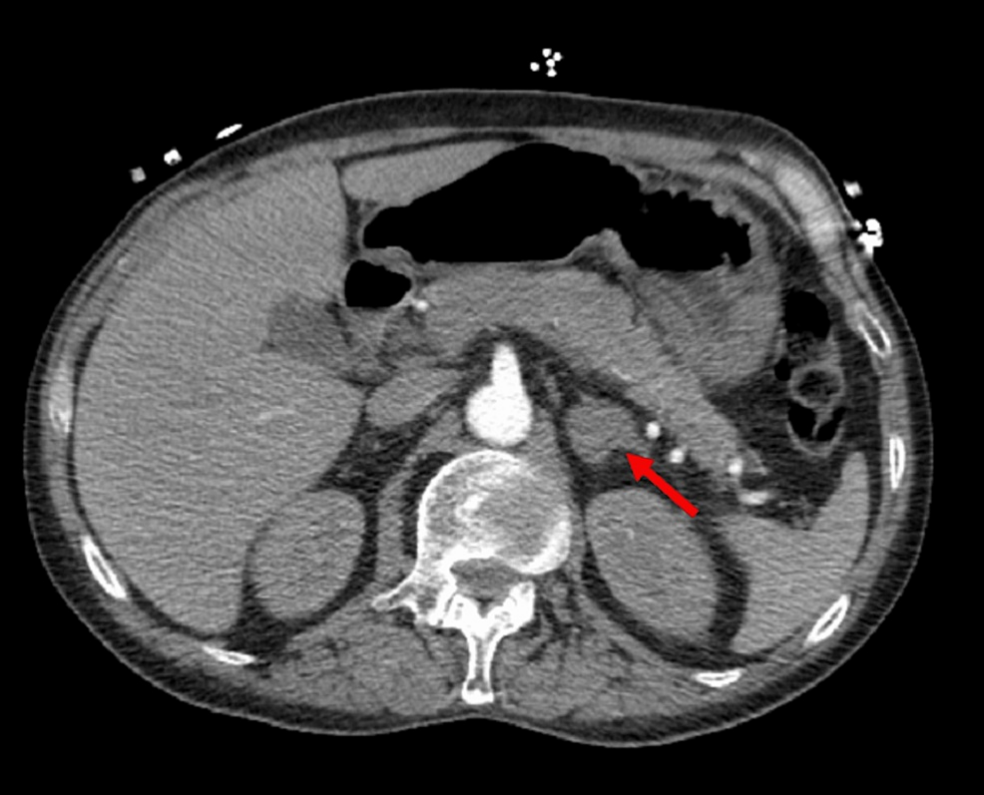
CT angiogram of the chest with contrast. CT chest, including parts of the upper abdomen, showing a new 2.7 cm enhancing left adrenal nodule not seen on his initial CT abdomen eight weeks prior, suspicious of metastasis. CT: computed tomography.
